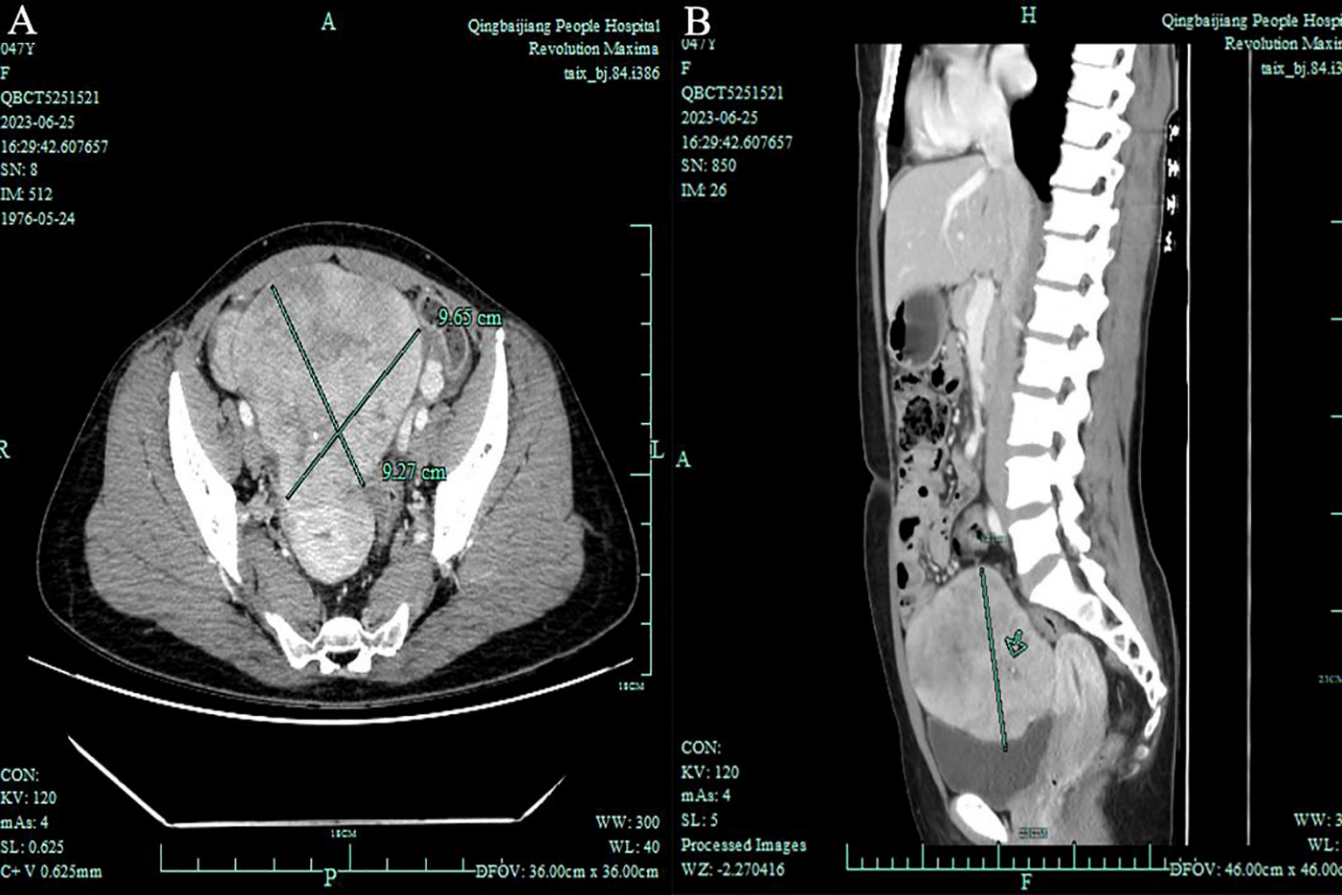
Total Abdominal Contrast CT Imaging: (A) Axial view - Demonstrates a pelvic space-occupying lesion measuring 8.6 × 9.7 × 9.3 cm, with indistinct posterior margins relative to the uterus. (B) Sagittal view - Highlights the lesion’s extent within the pelvic cavity without evident metastasis to the omentum or para-aortic lymph nodes.
